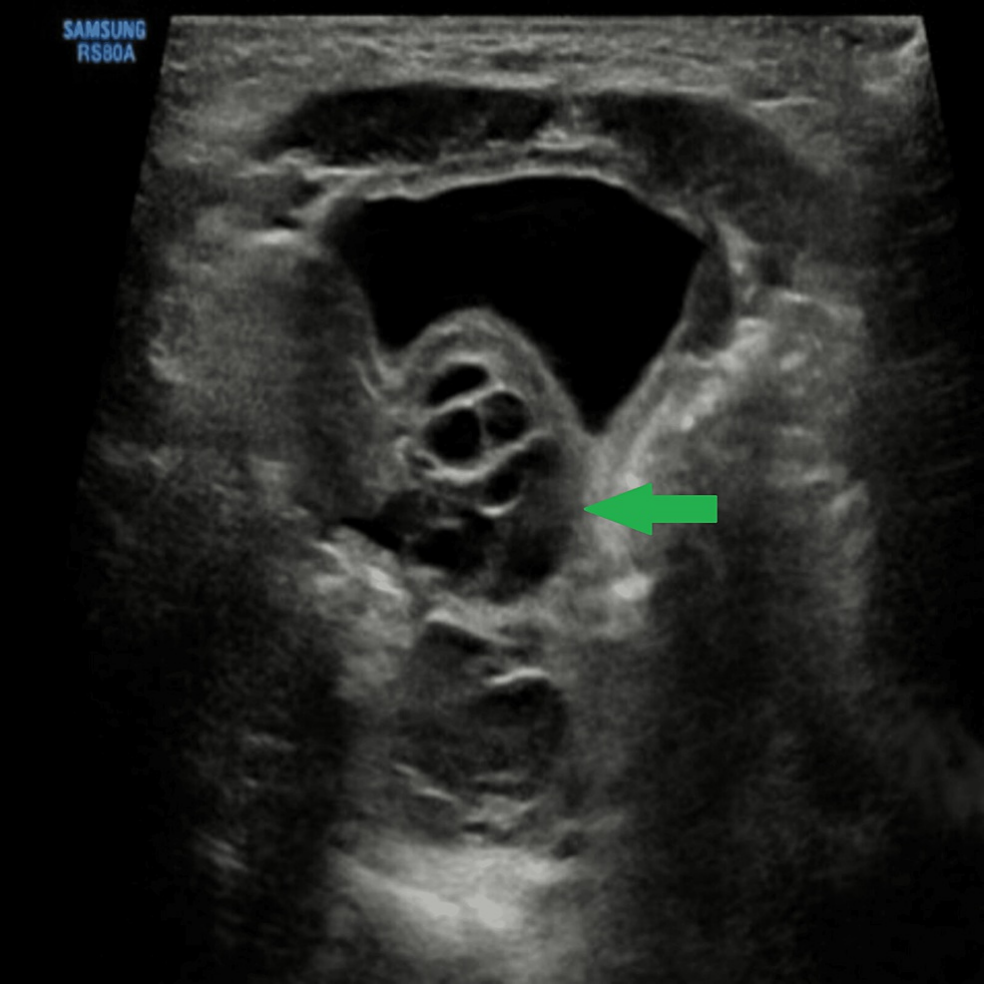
On B mode ultrasonography, multiple cystic areas (green arrow) are seen posterior to the urinary bladder showing the internal extension of the sacral lesion.
